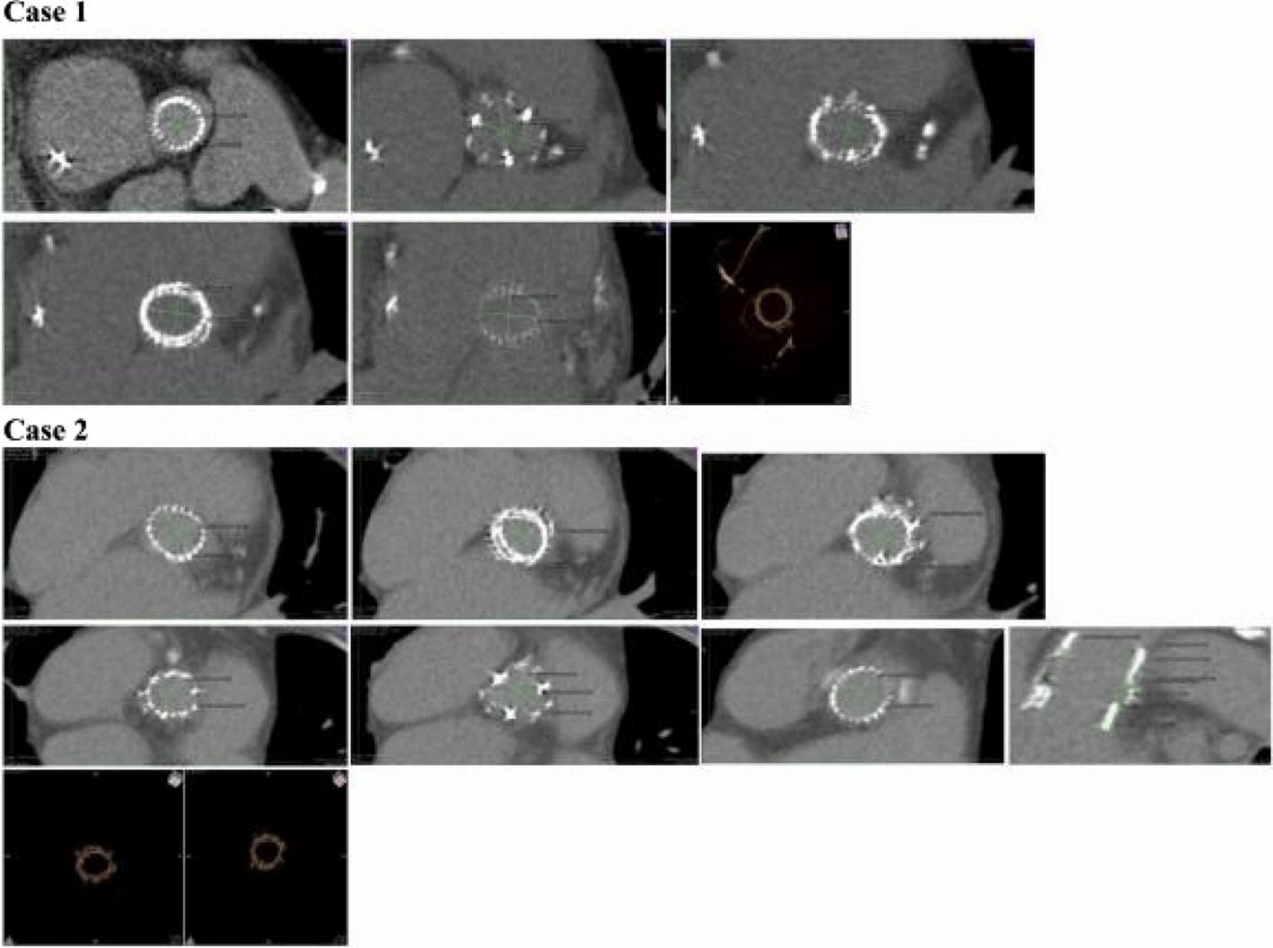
CT follow-up of Cases that received SUAVR with the Perceval image series obtained with the OsiriX™ providing us a pseudo-realistic three-dimensional depiction of the human body highlighting the aortic bioprosthesis in situ with different views at different levels of the valve, with the actual measurements taken on an appropriately reformatted CT scan
